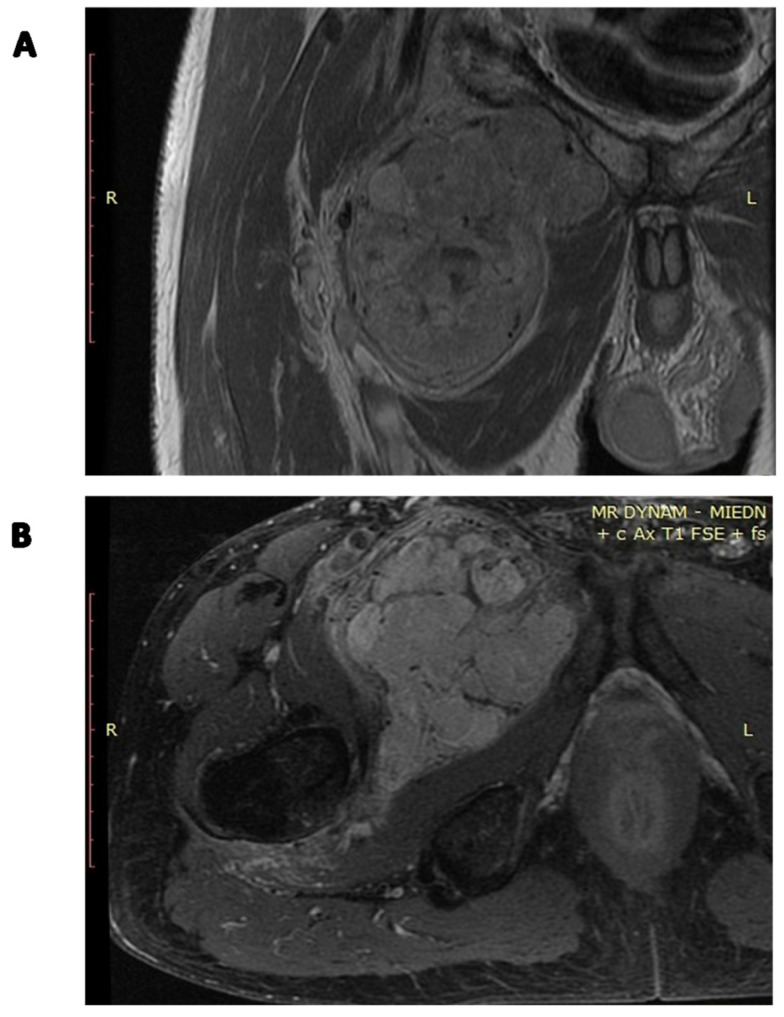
Preoperative radiotherapy contrast-enhanced MRI image of the SFT of the right groin: (A) coronal image; (B) axial image.
